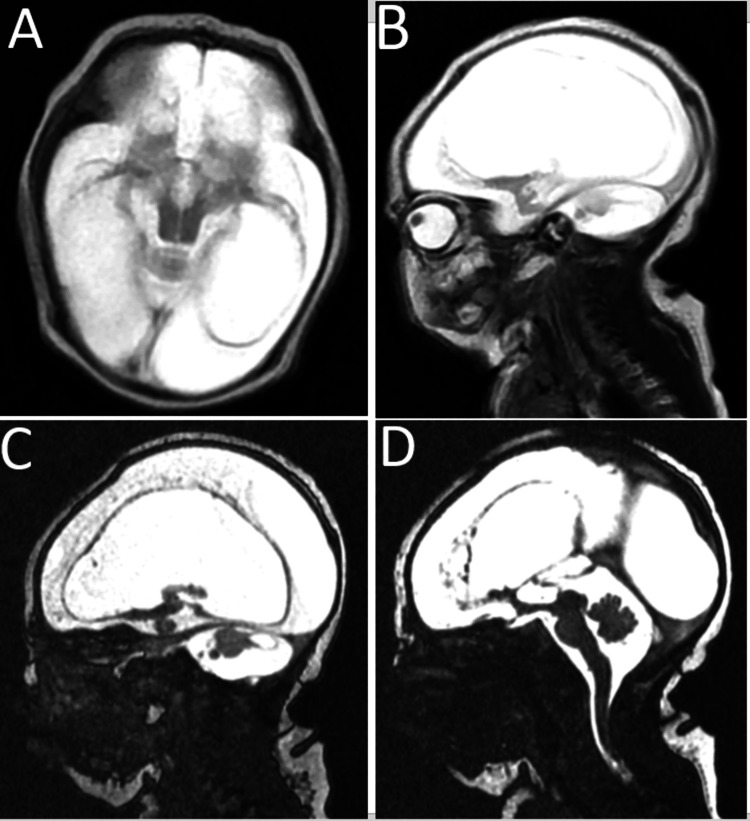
The MRI of the brain Axial (A) and sagittal (B) slices of a T2-weighted brain MRI show that the cerebral parenchyma and basal ganglia are almost entirely replaced by fluid isointense to CSF, with the persistence of a thin cortical rim. Sagittal image (C) shows atrophied cerebellar hemispheres with posterior cystic lesions containing fluid isointense to CSF. Sagittal image (D) shows a brainstem and vermis with normal morphology and signal.
